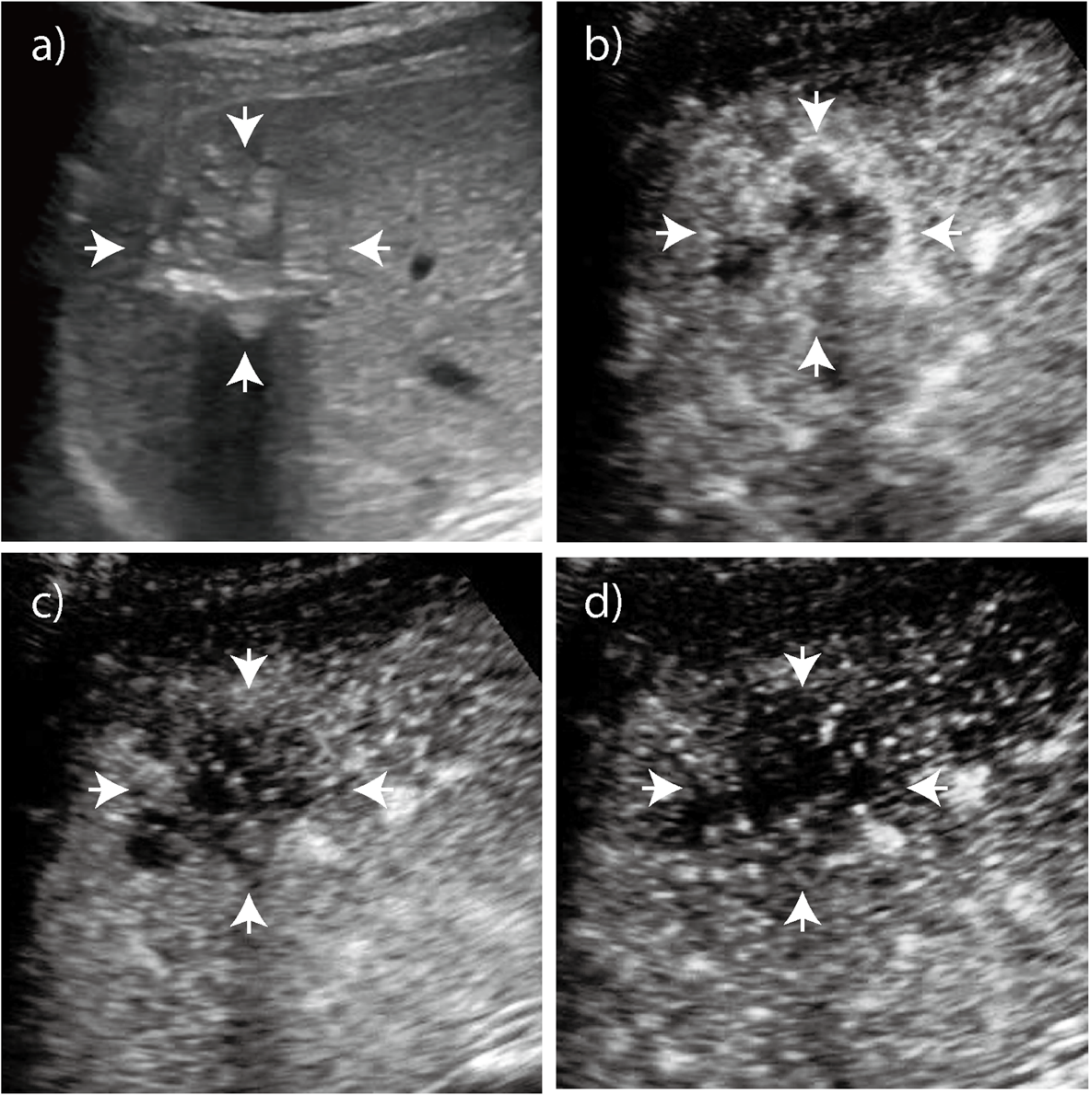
Hepatic alveolar echinococcosis (HAE). a Baseline ultrasound image shows a nodule with mixed echogenicity in the right lobe of the liver that is 5.0 cm in diameter. b On CEUS, arterial phase image obtained 15 s after administering the contrast agent shows diffuse heterogeneous hyperenhancement of the lesion. c - d Portal and late phase images obtained at 43 s and 131 s after administering the contrast agent, respectively. The nodule is hypoechoic with respect to the surrounding liver
